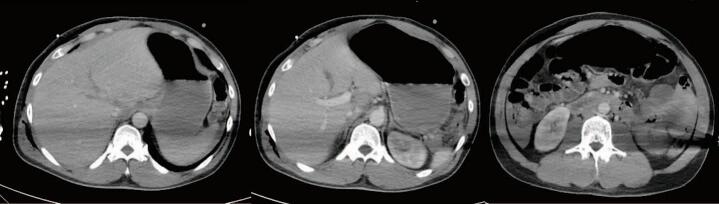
CT Abdomen Pelvis on Hospital Day 10 CT Abdomen and Pelvis on HD 10 demonstrated stellate liver laceration with healing and decreased hemoperitoneum.
